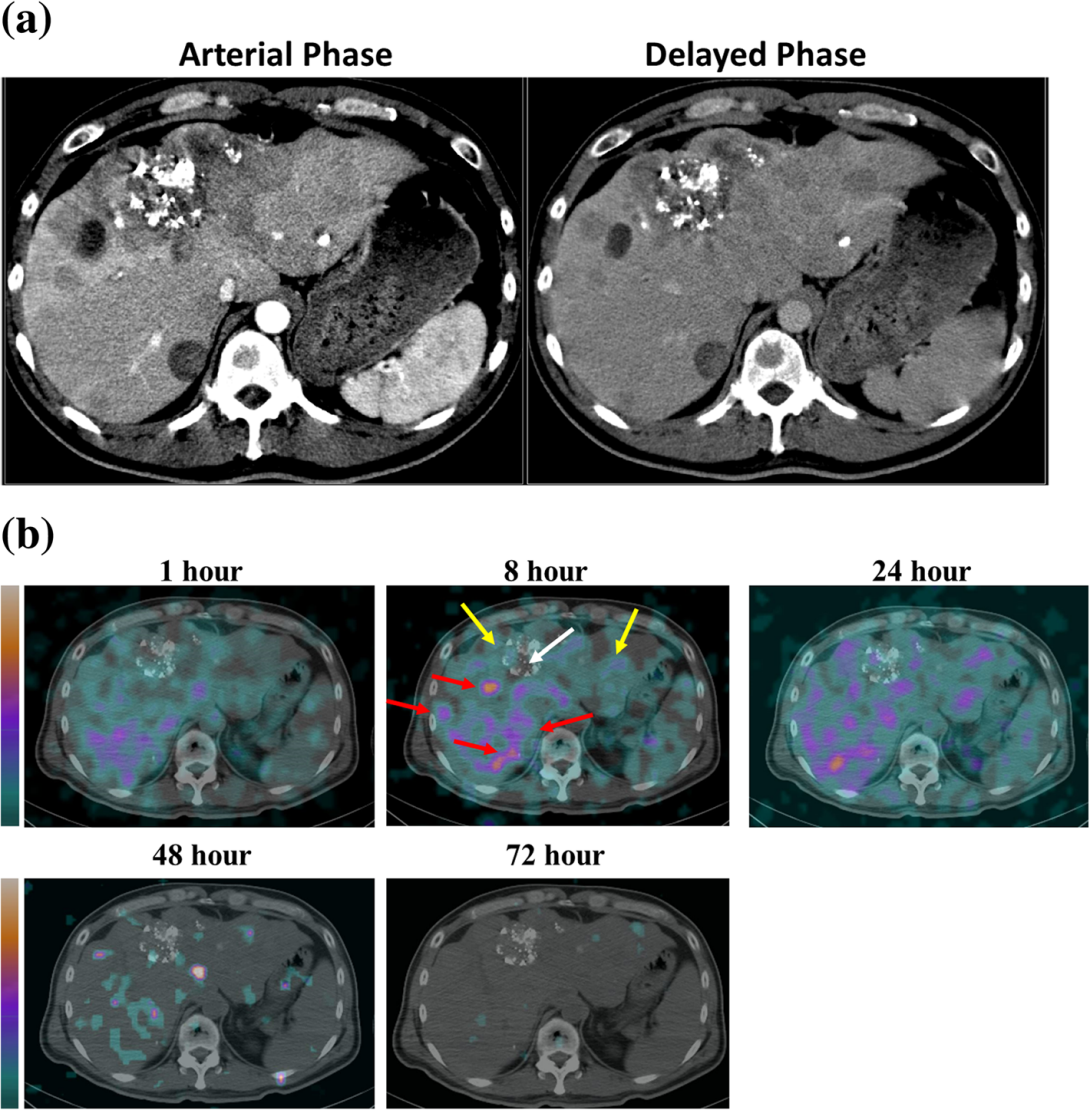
a Contrast CT image of a liver cancer patient (Pt. 3) with pulmonary and bone metastases displayed lipiodol retention resulted from transarterial embolization and multiple confluent nodular and mass lesions at both lobes of liver. b
